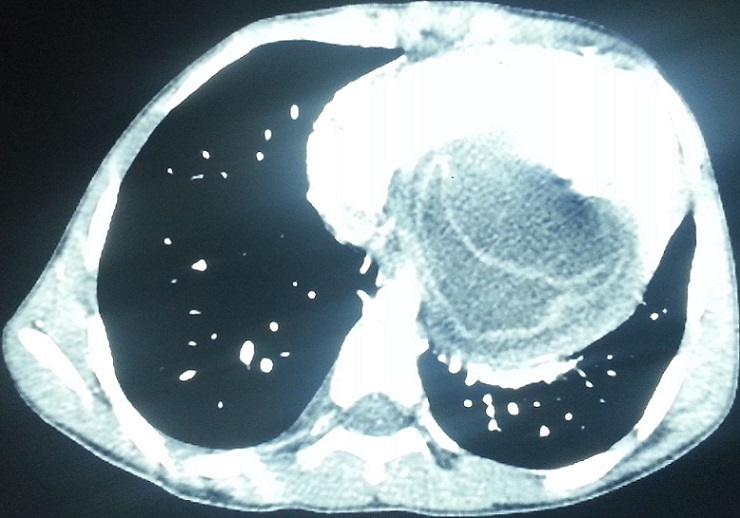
Thoracic helical CT scan in axial acquisition after injection of contrast material showing a pericardial cystic lesion next to right cavities especially the right ventricle, a detached layer is seen within the lesion, which compresses the ventricular myocardium
